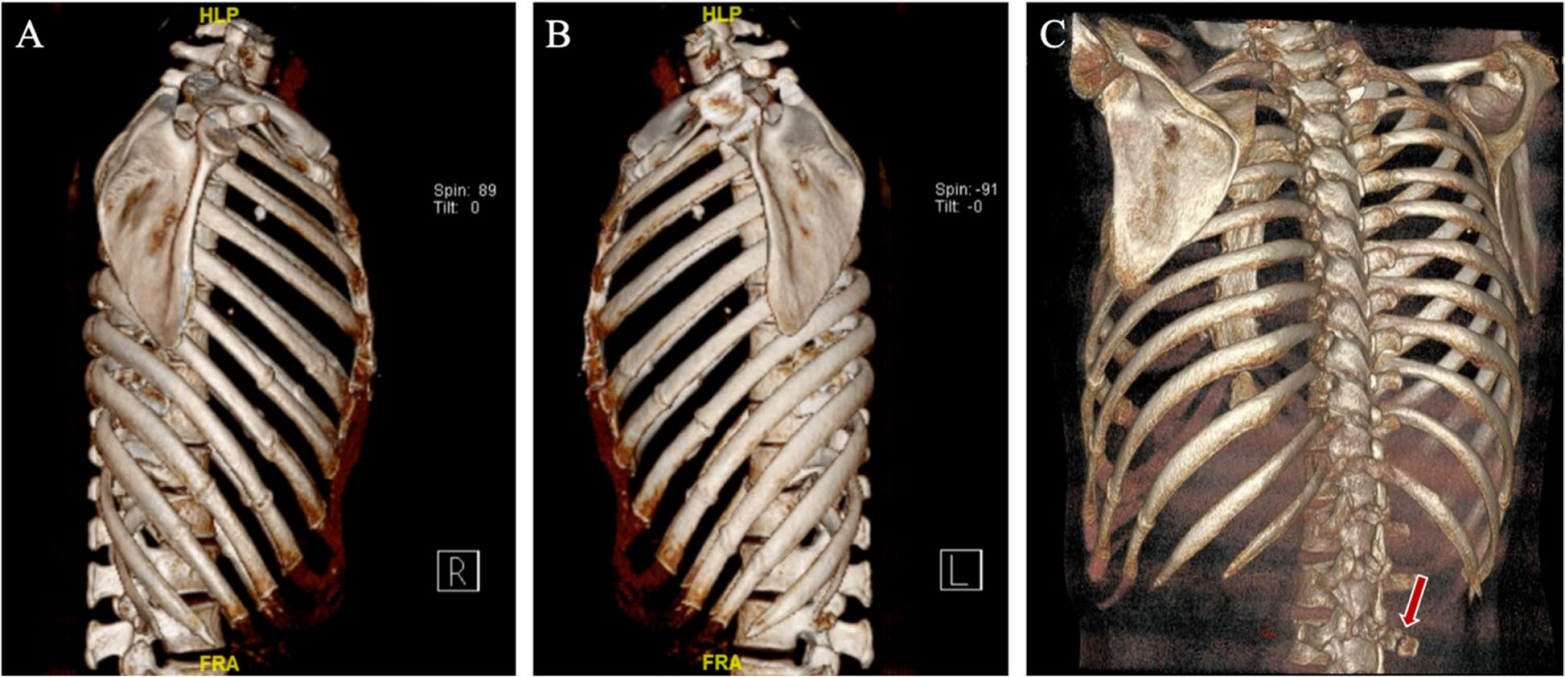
CT reconstruction of the rib cage and vertebrae reveals fractures and formation of calluses. A Right rib cage (fractures in 5th-10th). B Left rib cage (fractures in 5th-10th). C Fracture in the right transverse process of L3. The red arrow indicates the site of fracture
